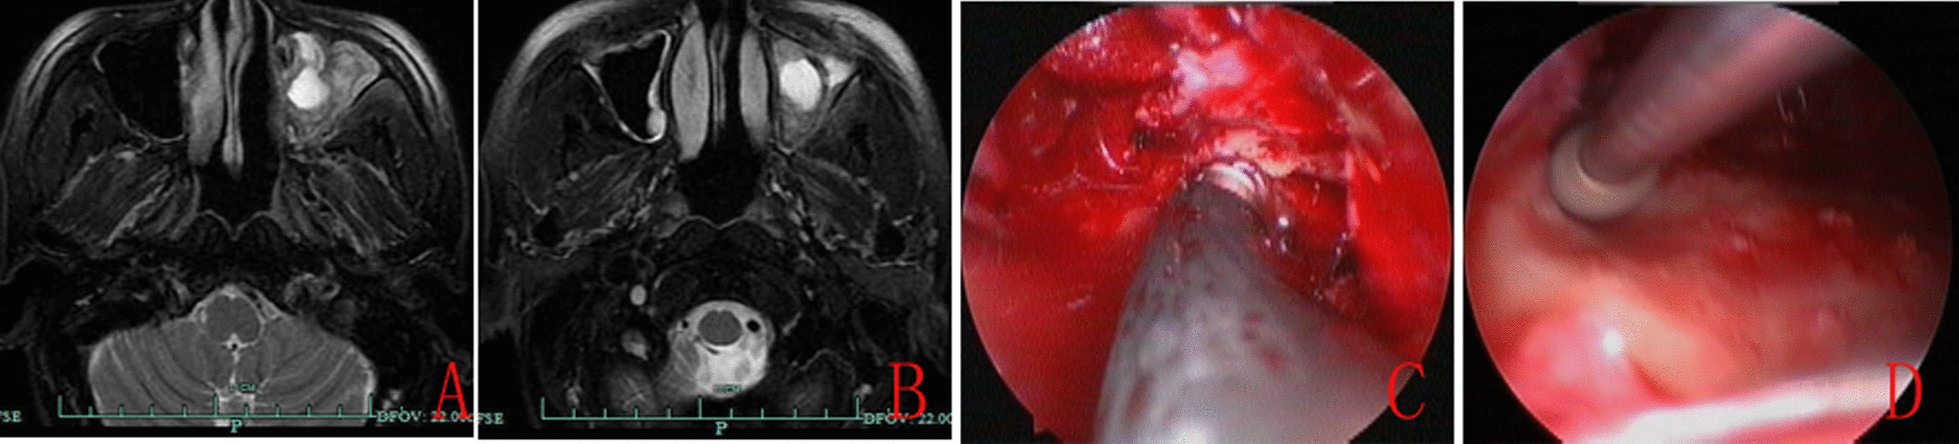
52 years-male. MRI revealed intensified soft tissue shade of left MS, the attachment sites of anterior wall (A and B), periosteum ablation (C), and bone drilling (D)
